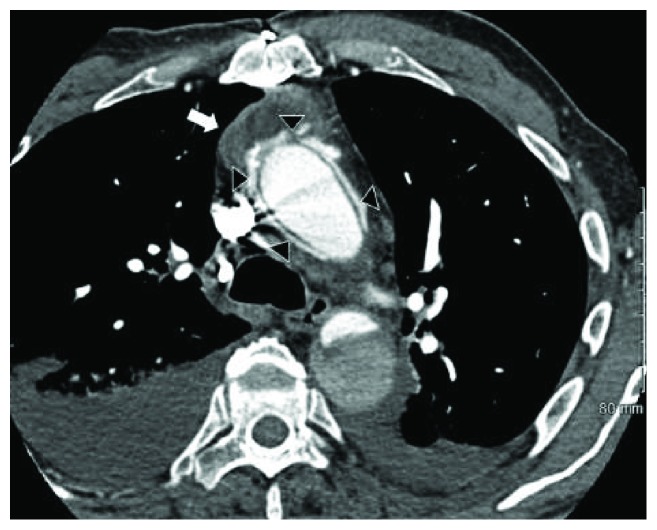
CT chest with contrast. White arrows show periaortic abscess in the ascending aorta.
